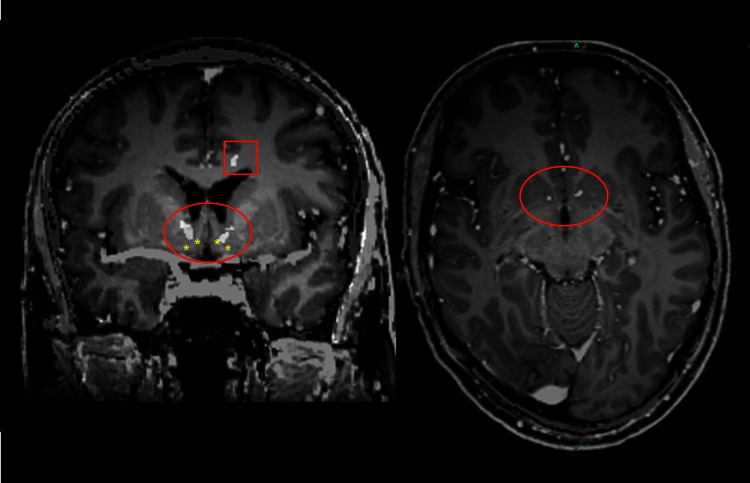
Distal electrode position in the NAC, depicted postoperatively with CT-fused presurgical T1–MRI in coronal and axial view (*red circles*). Both distal contacts of the quadripolar electrodes were placed in the NAC as intended, the third contact within the transition area to the medial border of the abutting internal capsule and the highest, i.e. the fourth contact at a point in the most medial part of the capsule or in the transition area to the caudate. *Asterisks* mark the medial and ventral border of the NAC region. The *red square* depicts an electrode lead to the left anterior nucleus of thalamus
